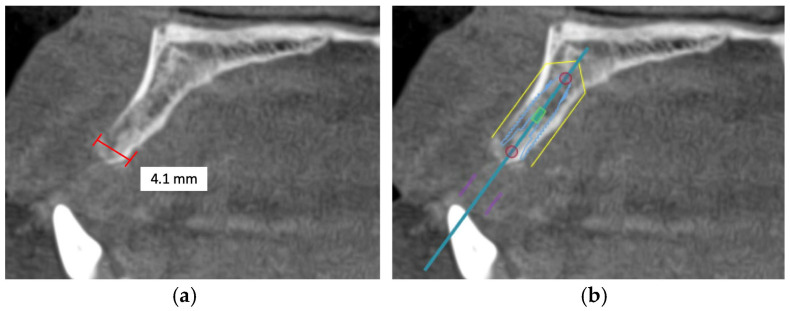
This CBCT image demonstrates a limited maxillary alveolar bone width pertinent to implant planning: (a) the alveolar ridge exhibits reduced width, which may be inadequate for the demands of implant placement; (b) given that the intended implant position exceeds the alveolar ridge width, horizontal bone augmentation is necessary.
